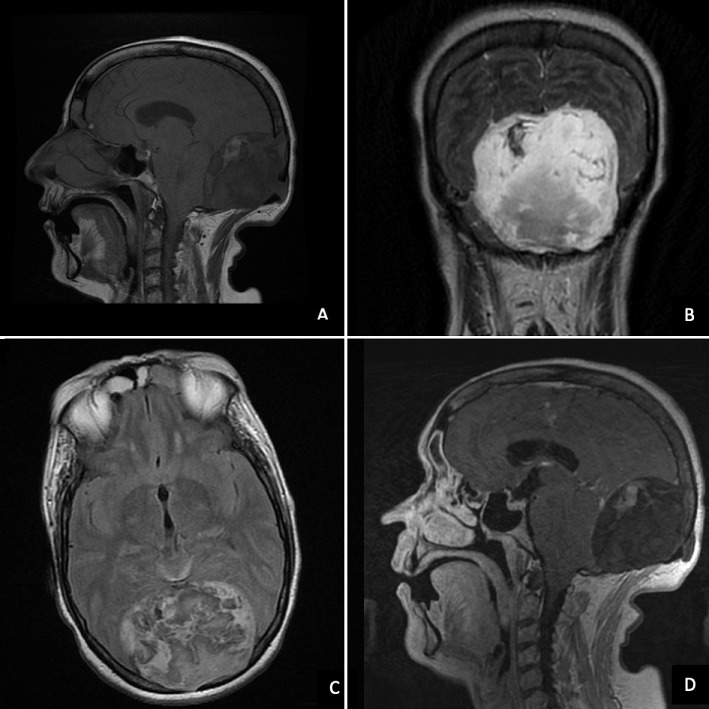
IRM cérébrale montrant un processus lésionnel extradural médian de la FCP en hyposignal T. (A) hypersignal T2, (B) hétérogène en FLAIR, (C) avec rehaussement périphérique modéré, (D) en rapport avec un kyste épidermoïde extra-axial
